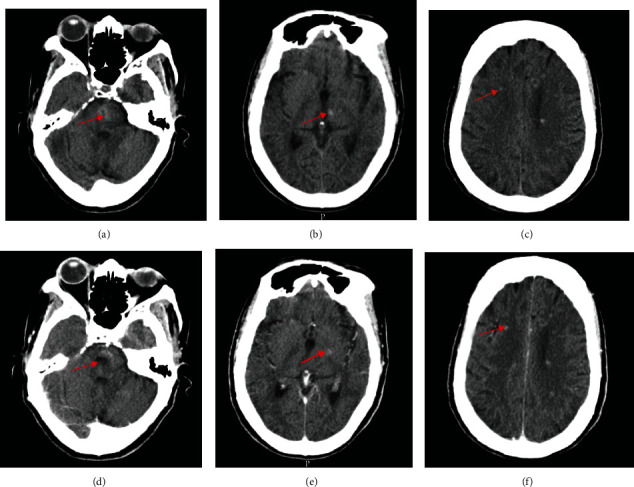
Axial (a–c) unenhanced and (d–f) enhanced cerebral computed tomography images showing multiple, diffuse hyperdense lesions in the infratentorial (pons), basal ganglia, and other supratentorial regions of the brain (red arrows) suggesting multiple cerebral cavernomas.
